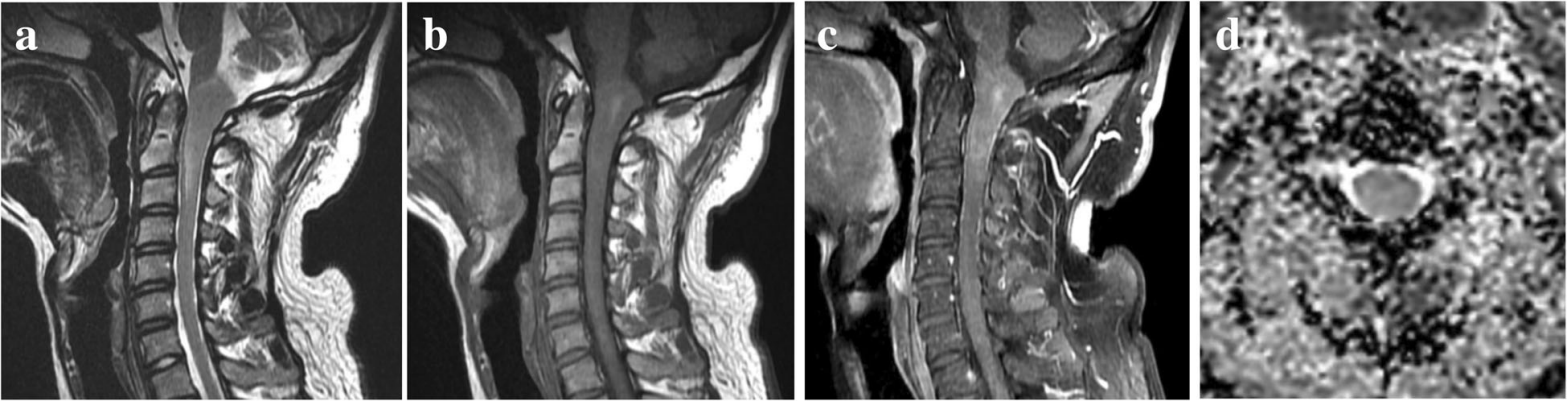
Cervical PA. a, Preoperative sagittal T2-weighted MR image shows a solid lesion in the cervical region presenting slightly hyperintense signal. Mild peritumoral edema is seen. b, Sagittal T1-weighted MR image shows a high signal intensity region corresponding to hemorrhage in the tumor. c, Sagittal contrast-enhanced T1-weighted MR image shows patchy enhancement of the tumor. d, ADC map shows the solid component shows increased water diffusion (ADC value = 1.32 × 10^− 3^ mm^2^ /s)
